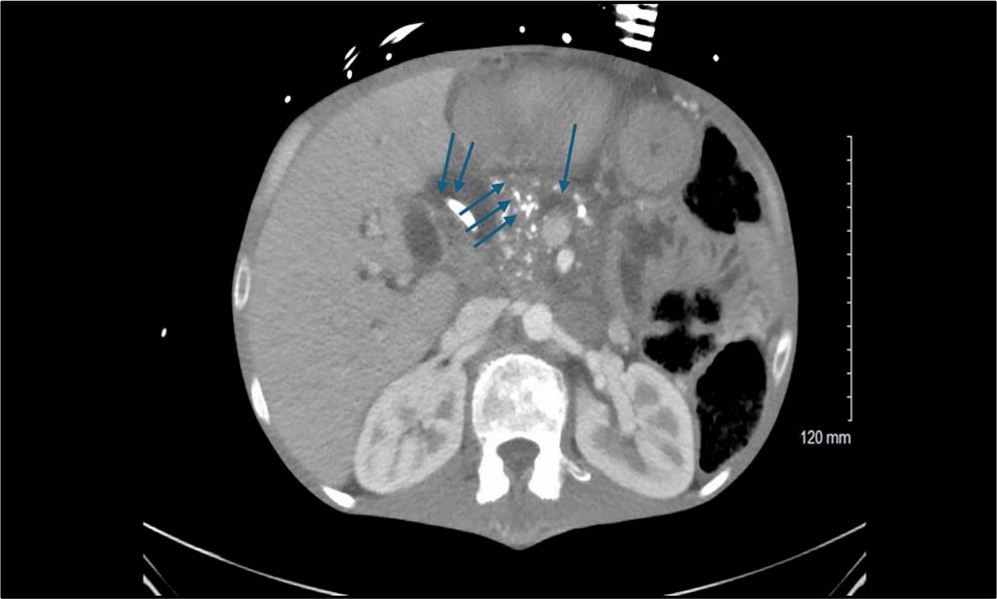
Preoperative computed tomography scan of the abdomen showing the calcified head of the pancreas (triple arrows), dilated pancreatic duct (single arrow), and dilated common bile duct (double arrows).
